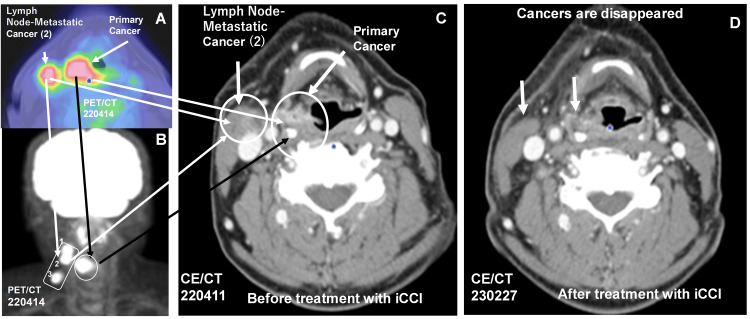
Complete response of locally advanced hypopharyngeal squamous cell carcinoma by treatment with an innovative combination cancer immunotherapy (iCCI) comprising radiotherapy, immune checkpoint inhibitors (ICIs), and dendritic cell (DC) vaccine pulsed with helper/killer hybrid epitope long peptide The patient was diagnosed as inoperable stage IVA hypopharyngeal squamous cell carcinoma with 2 cm primary and three lymph node (LN) metastatic cancers by PET-CT (A, cancer lesions were indicated by arrows; B, primary cancer is indicated by black arrow with circle and three LN lesions (1, 2, 3) are indicated by a white arrow with rectangle] and CECT; C, primary and LN metastatic cancer (2) lesions were indicated by white arrows with circles). The outcome of iCCI treatment against advanced HNSCC was diagnosed by CECT on August 18, 2022 (D), and it was demonstrated that all cancers, including the primary and three LN metastatic cancers on the right side of the neck, completely disappeared (D, the disappearance of cancer 2 were indicated by arrows and the disappearance of other two cancers (1 and 3) are indicated in Figure 3) by treatment with iCCI. No recurrence has been confirmed by CECT on February 27, 2023, and October 16, 2023.
